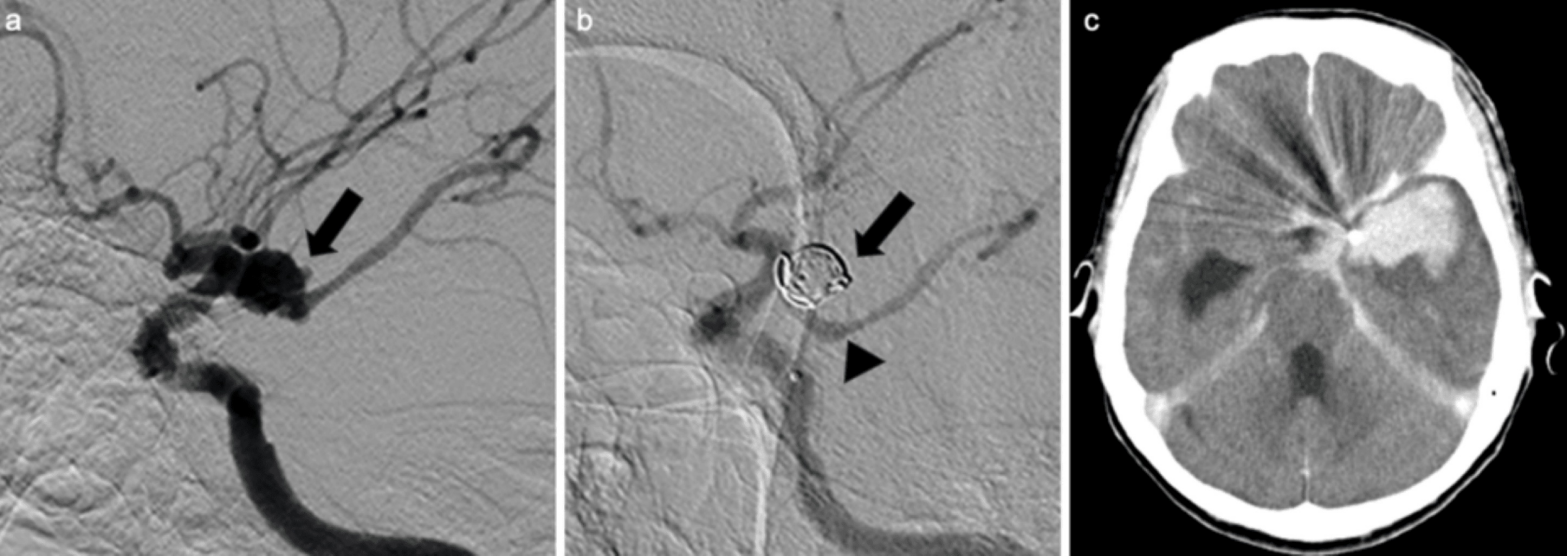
Coil embolization procedure (a) Angiography (left anterior oblique view) before coil embolization showing an aneurysm at the left internal carotid-posterior communicating artery bifurcation with a bleb (arrow). (b) Angiography (left anterior oblique view) after coil embolization confirming successful embolization within the aneurysm (arrow), with maintained blood flow through the posterior communicating artery (arrowhead). (c) CT scan immediately following coil embolization showing no increase in the intratemporal hematoma.
